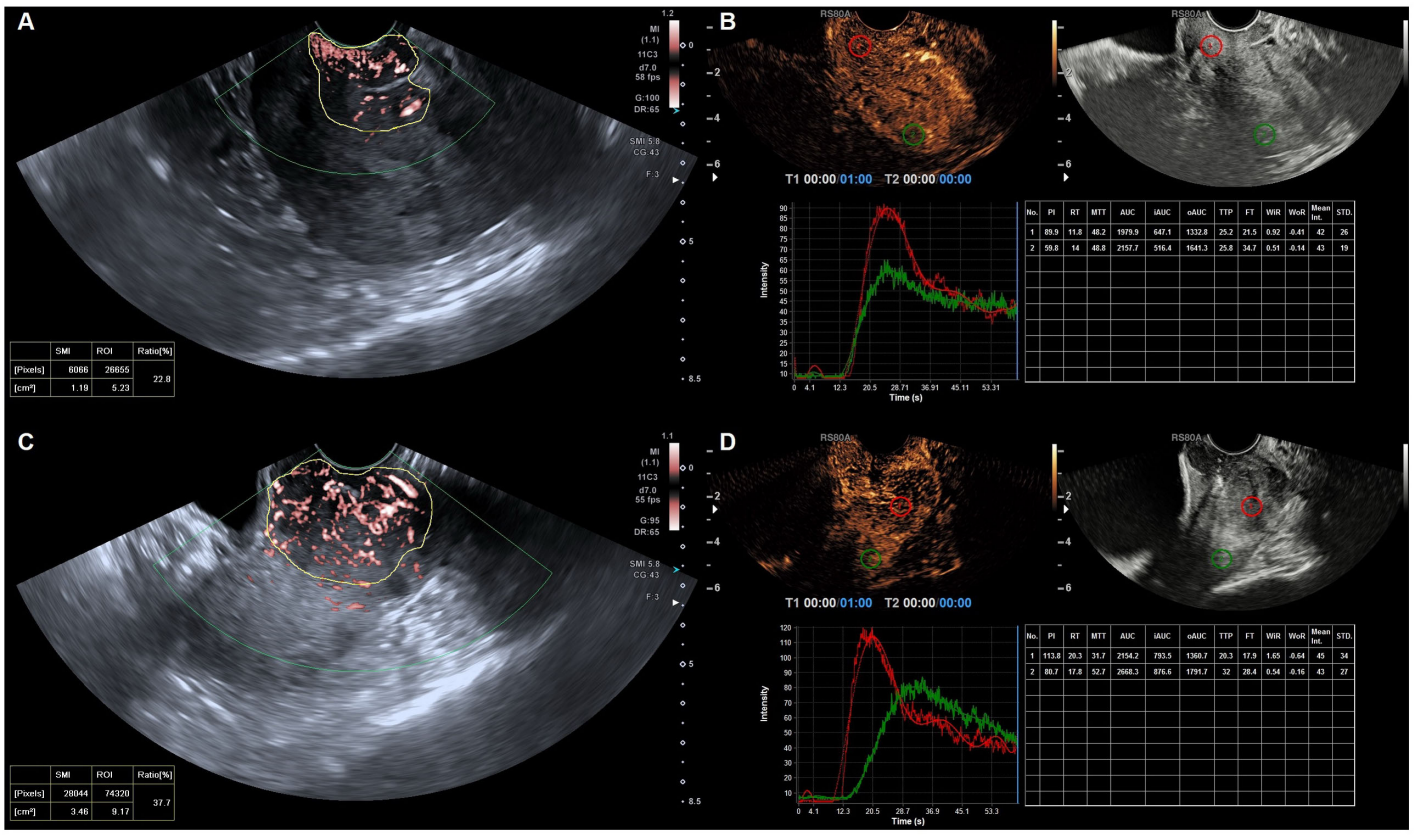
Quantified superb microvascular imaging (qSMI) and quantified contrast-enhanced ultrasonography (qCEUS) in cervical cancer of the different groups. (A) qSMI and (B) qCEUS in a 52-year-old with confirmed stage IIA1 cervical cancer followed by surgical treatment. (C) qSMI and (D) qCEUS) in a 56-year-old with confirmed stage IIIC1 cervical cancer followed by chemoradiotherapy. VI was obtained by manually delineating the boundary of the cervical lesion (in yellow) on the SMI images. Output TIC for cervical cancer (in red) and myometrium (in green).
